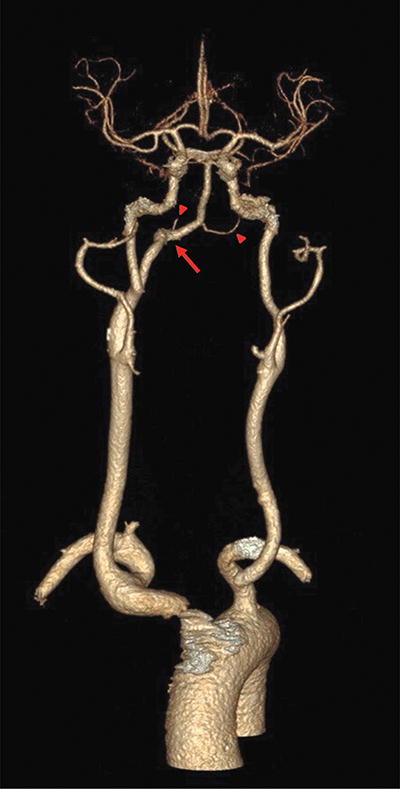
3D reconstruction of CT-angiography showing a right persistent hypoglossal artery (arrow) and hypoplastic vertebral arteries (arrowheads).
